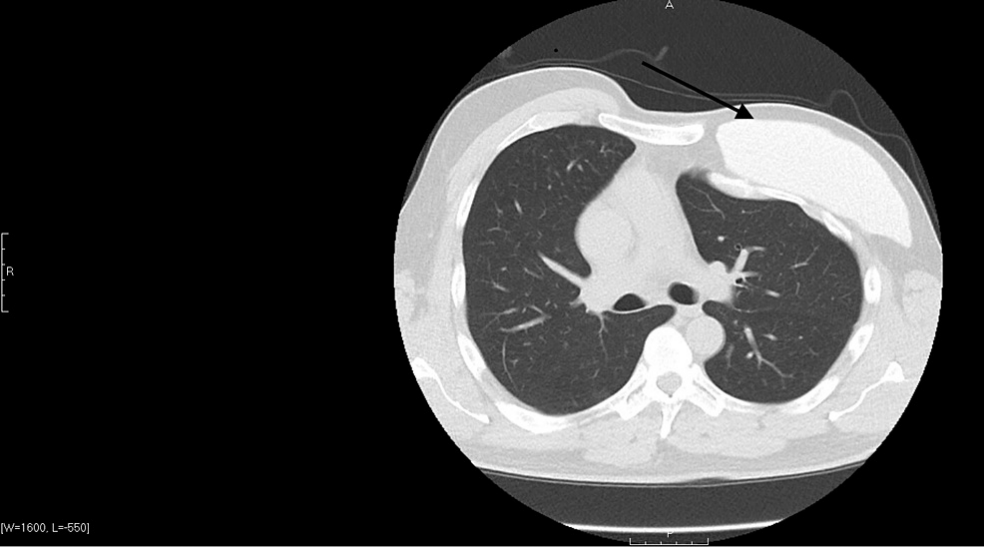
Chest CT without contrast demonstrating abnormal left chest wall musculature (depicted by the arrow), with a cosmetic left breast implant.
